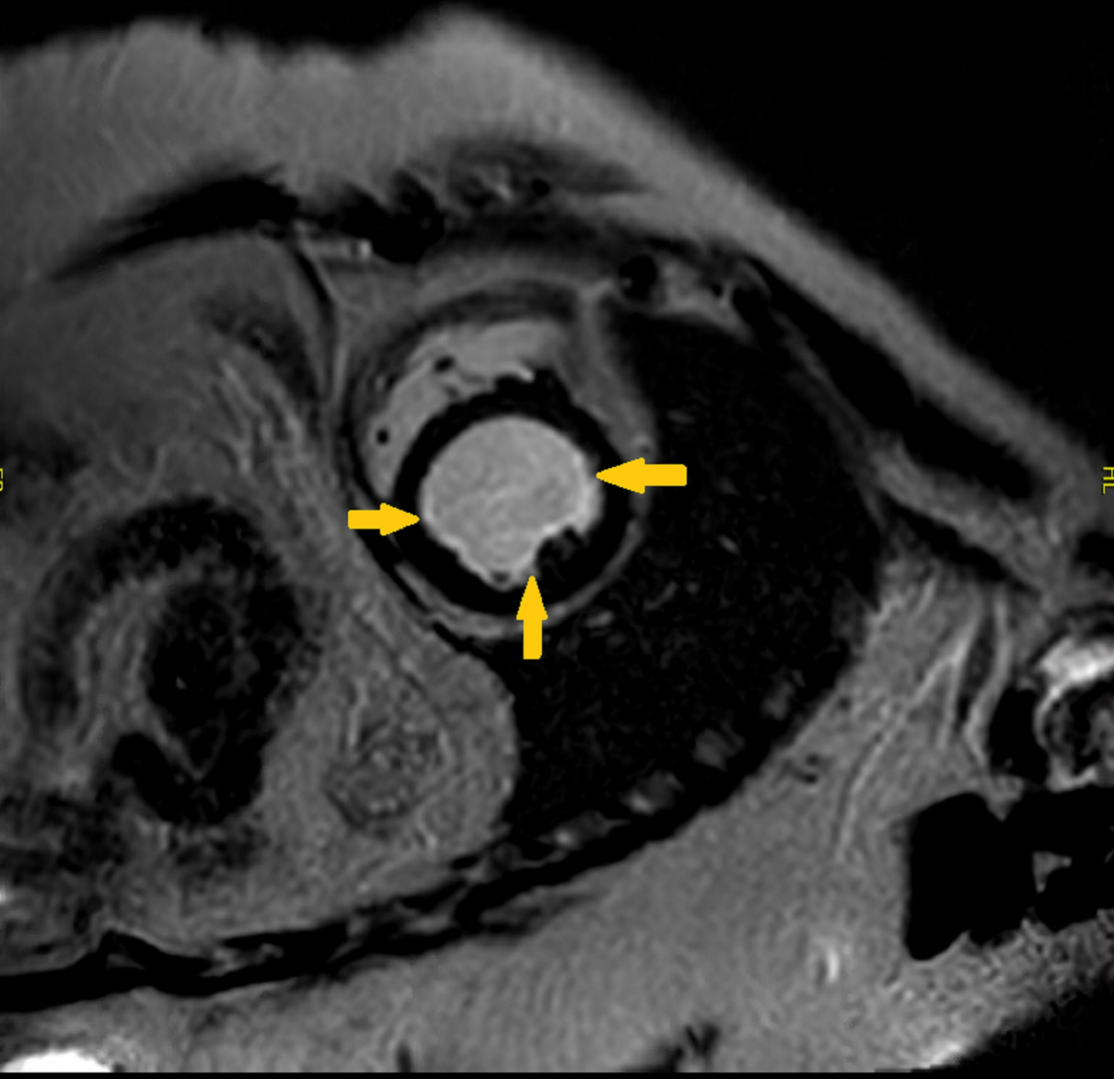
Cardiac MRI Cardiac MRI in short axis view showing rim of subendocardial delayed enhancement (arrows)
